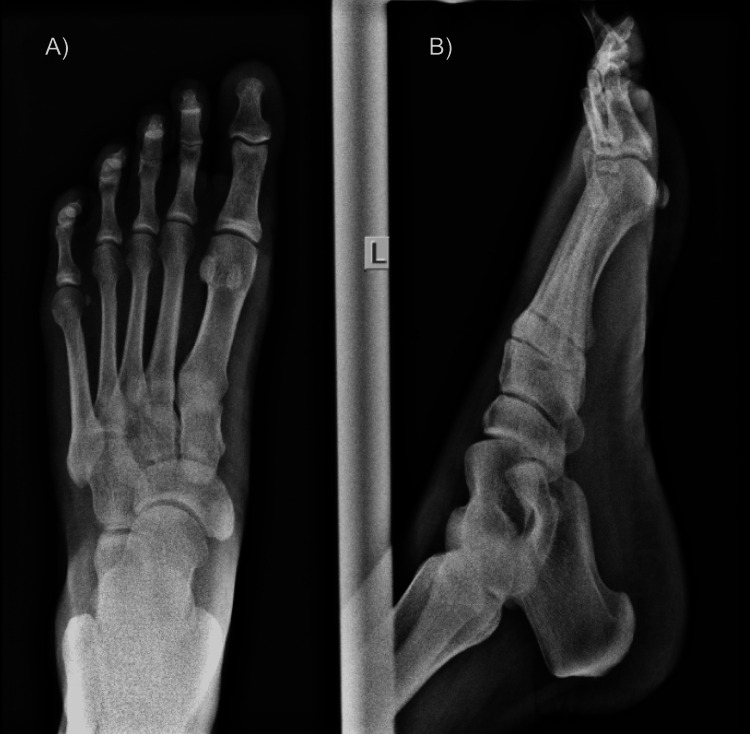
Left foot X-ray images in dorsoplanter (A) and lateral (B) views
